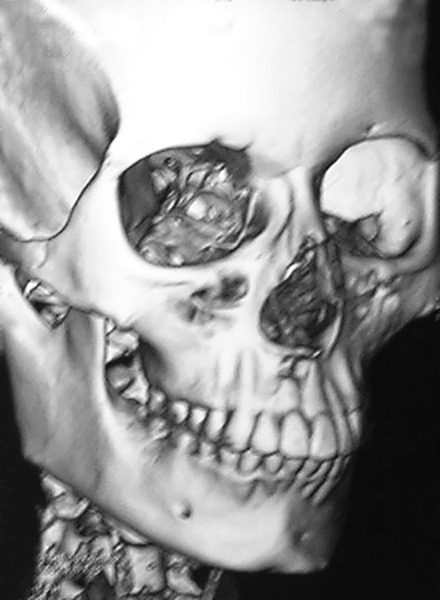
Compare the pre- and postsurgery CT. See the difference between locations of strangely positioned area
